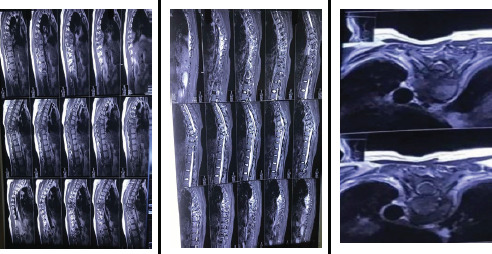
A) T1-weighted MRI sagittal section. B) T2-weighted MRI sagittal section C) The transverse section at the T8 and T9 levels demonstrates that pedicle fractures cause a separation between the posterior and middle columns, which prevents cord injury by maintaining the integrity of the spinal canal.
